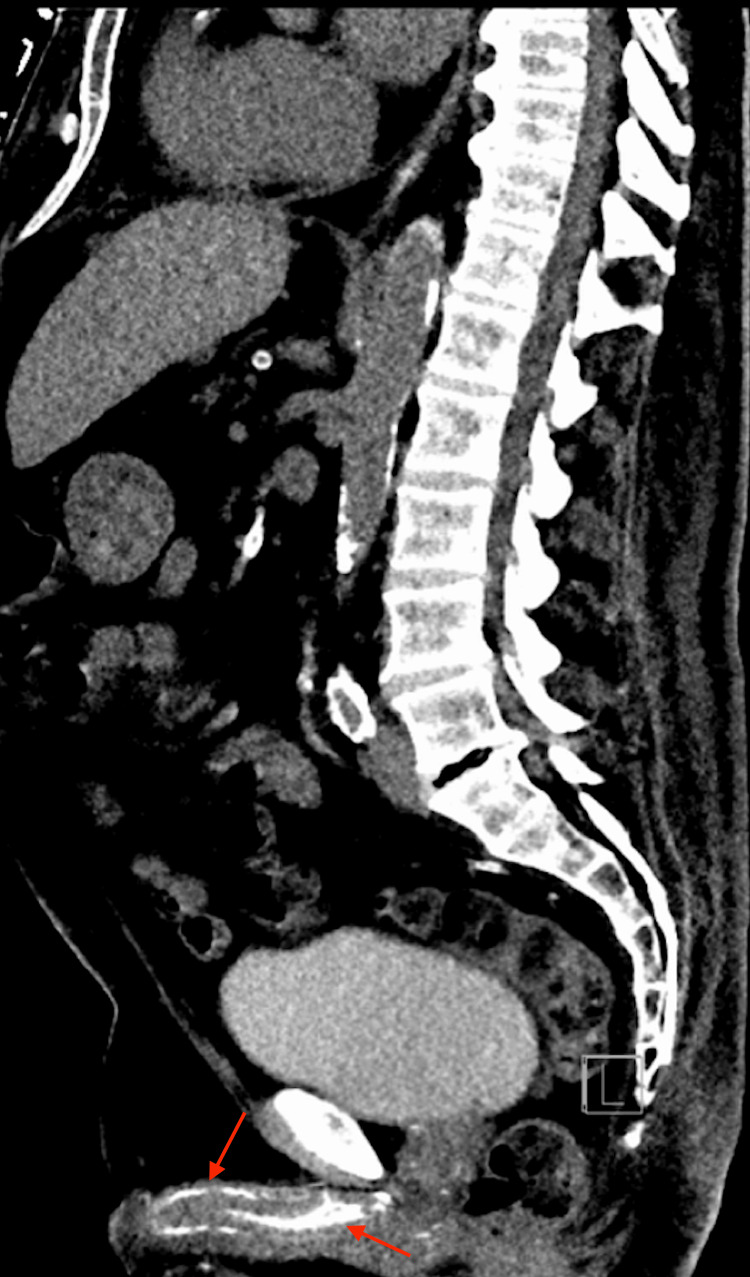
CT Abdomen and Pelvis without contrast, sagittal view demonstrating calcification within the penile vasculature.
